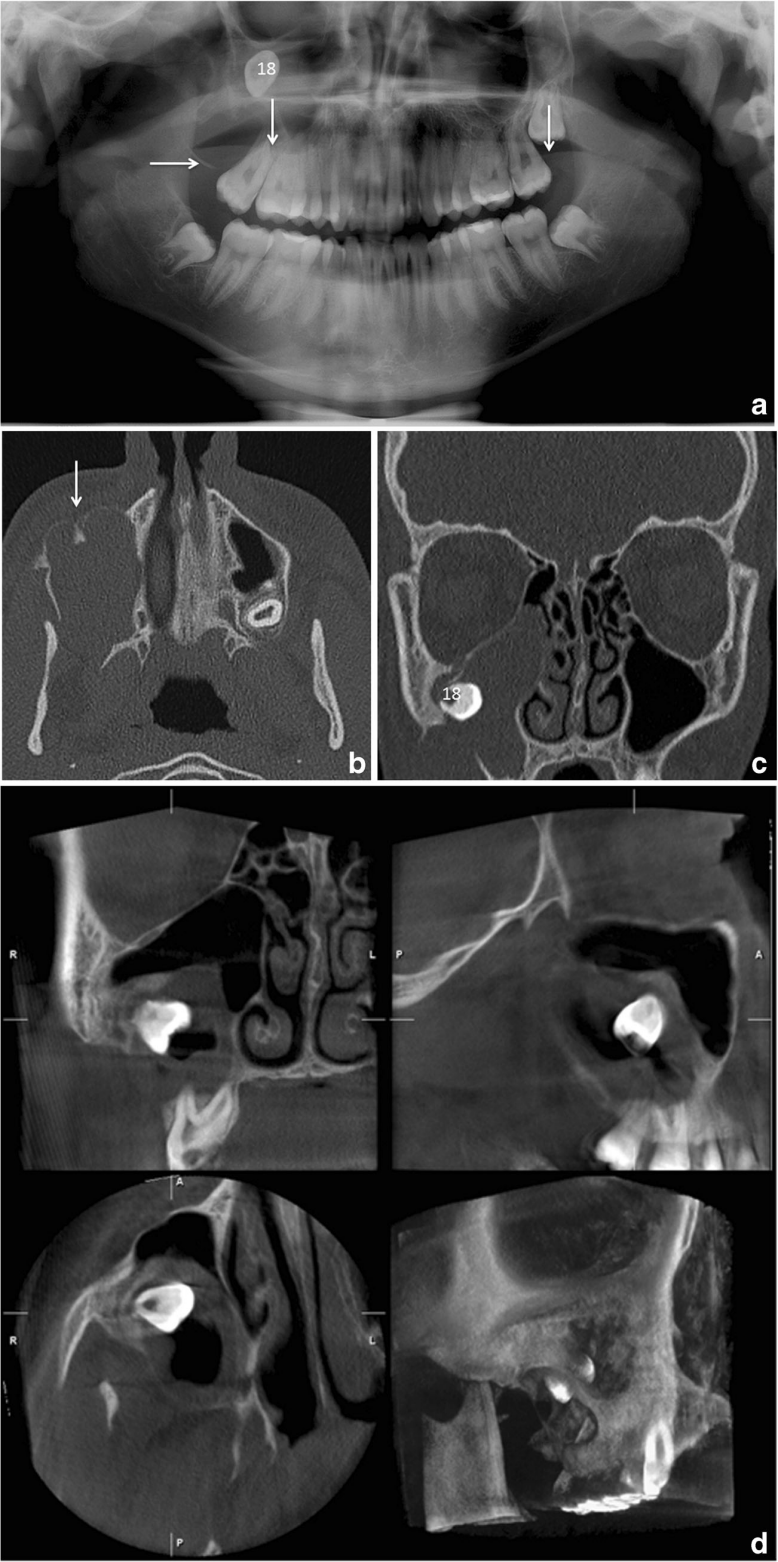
a PTG reveals cranial dislocation of d. 18 into the right maxillary sinus with a cystic lesion causing expansion of the sinus walls. Note the head positioning error in the PA plane; the patient has been positioned anterior to the image layer. Also the tongue (*vertical arrows*) is not against the palate. b Low dose MSCT axial and c coronal images show dislocated d. 18 surrounded by a large cystic lesion that causes expansion and perforation of the sinus wall. In the anterior wall of the sinus characteristic border scalloping of keratocystic odontogenic tumour (*KCOT*) is evident (*arrow*). d CBCT examination taken 5 months after the fenestration with concomitant biopsy of the lesion. The tumour was later operated
